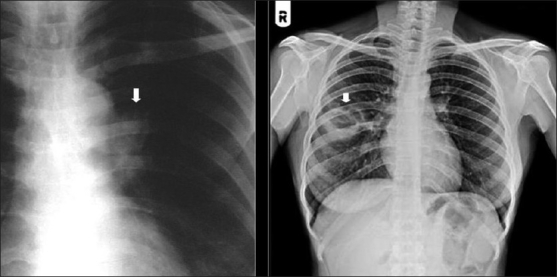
Nontuberculous/atypical Mycobacterium (NTMB). The chest X-ray (CXR) abnormalities resemble *Mycobacterium tuberculosis*, with focal consolidation CXR (left), diffuse patchy infiltrates and cavities (right). The features are, however, nonspecific and the diagnosis is often delayed
